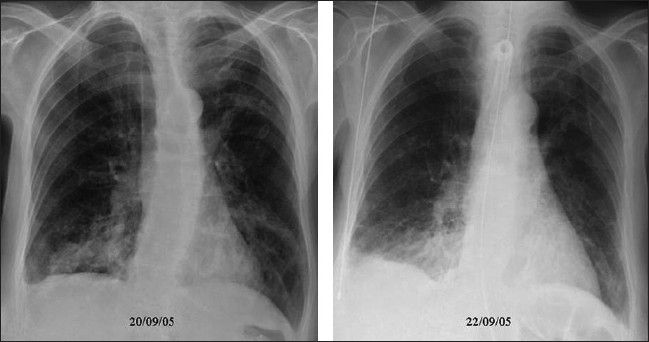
An AP chest radiograph of a patient with tracheostomy showing development of aspiration pneumonia at the right lung base
